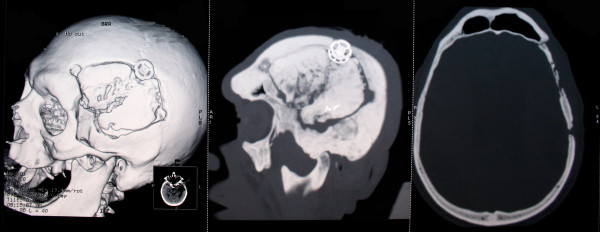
Preoperative CT scan. CT bone window shows partial osteolysis of the bone flap, due to osteomyelitic process.
